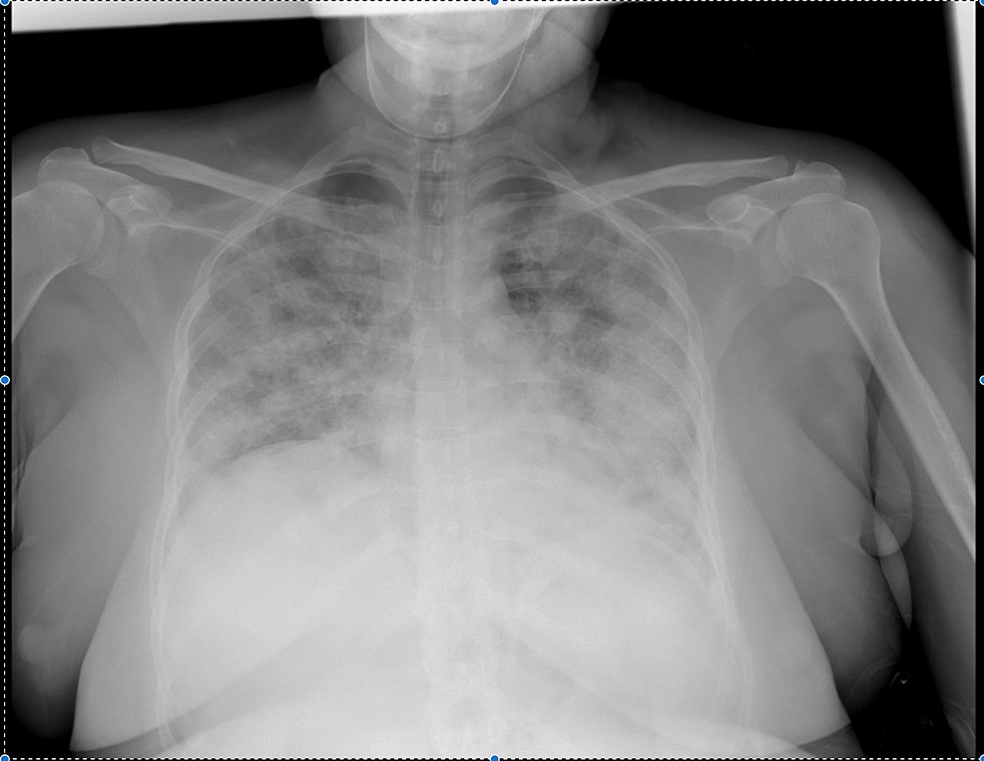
Chest X-ray PA view (bilateral diffuse opacities).
